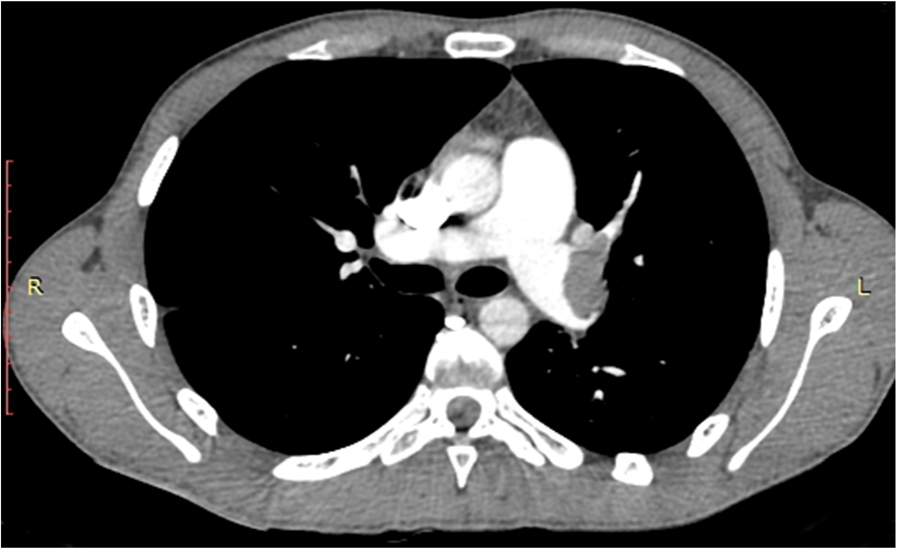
Preopoperative CT angiography showing massive LPA embolism with small emboli in the right upper branch of RPA.
